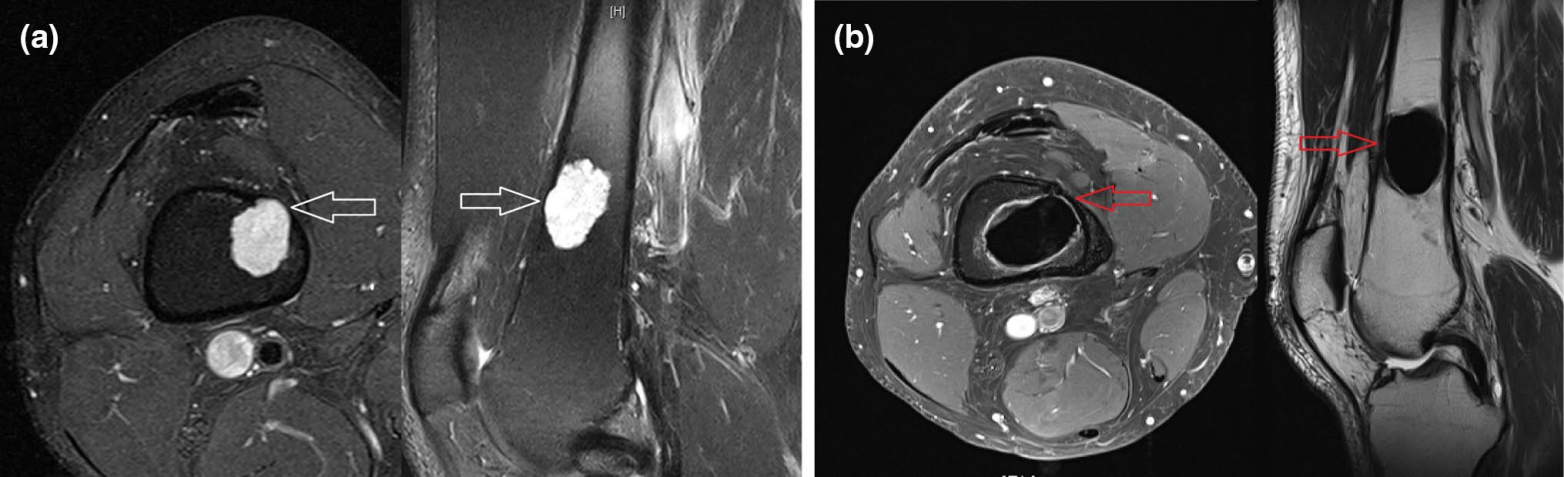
Preoperative MRI with endosteal scalloping and soft tissue extension (white arrows) of the lesion located in the metaphysis and diaphysis (a). Treatment was performed with intralesional excision and bone cement filling without additional osteosynthesis (b). Eighteen months postoperatively cortical bone grew over the initial scalloping zone and bone window (red arrows) resulting in a stable distal femur. The bone cement filling is regularly surrounded by edema (white margin around the filling), which must be distinguished from local recurrence (color figure online)
